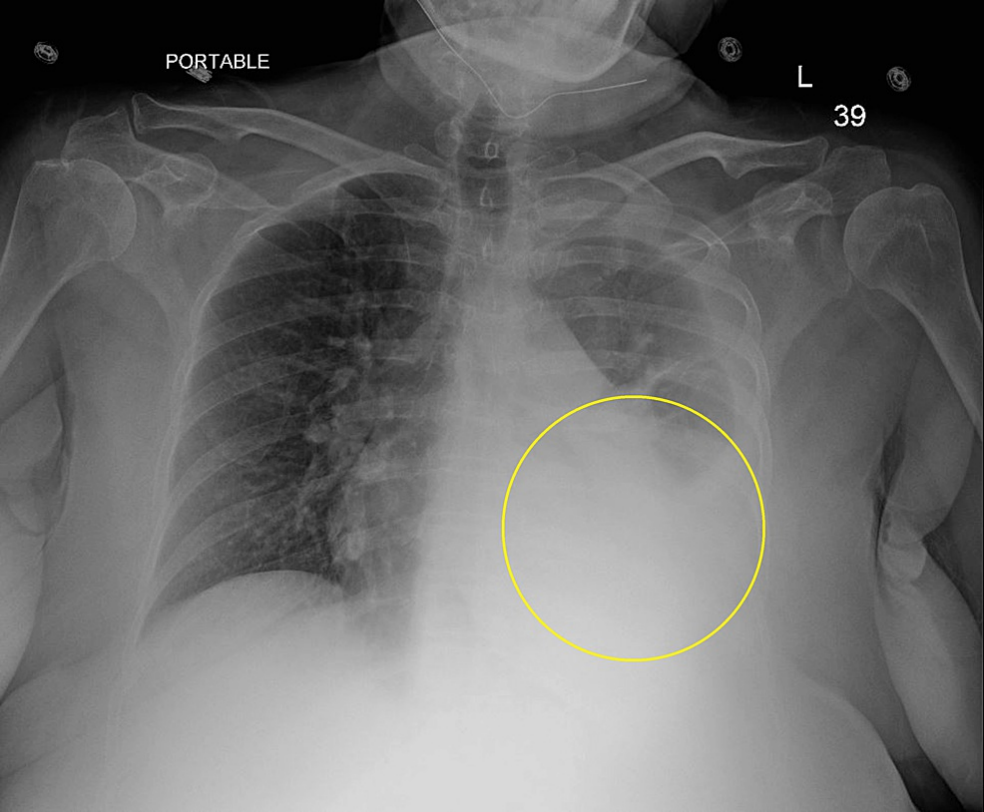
Chest X-ray Yellow circle: pleural effusion
